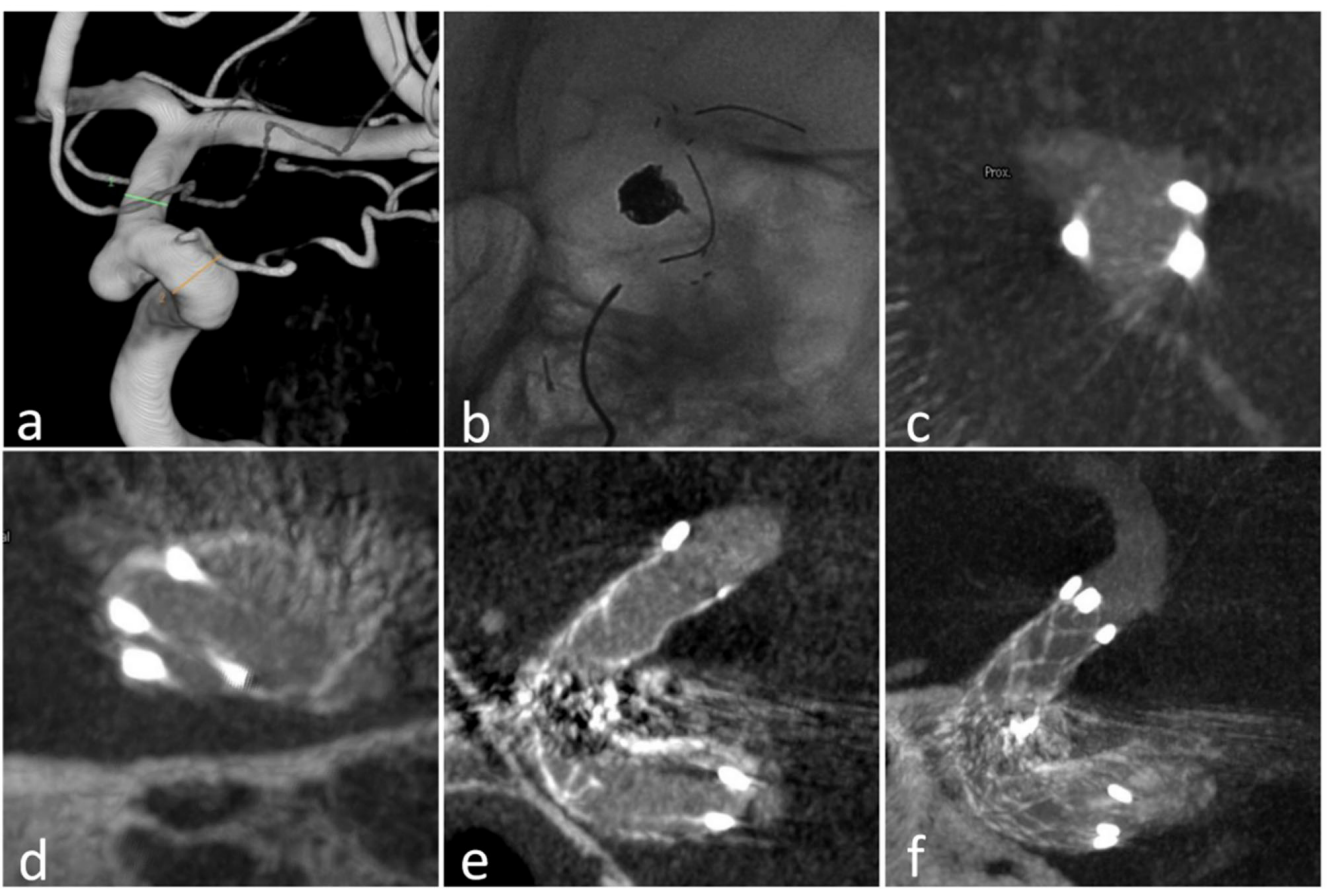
Case 18: (a) the aneurysm at the ophthalmic arterial segment of ICA; (b) the stent (23 mm) in the ICA; (c) the proximal markers were asymmetrical; (d) the distal markers were symmetrical; (e) sagittal view of the stent in Xper CT; (f) correct stent wall position of the deployed stent with larger thickness in Xper CT.
